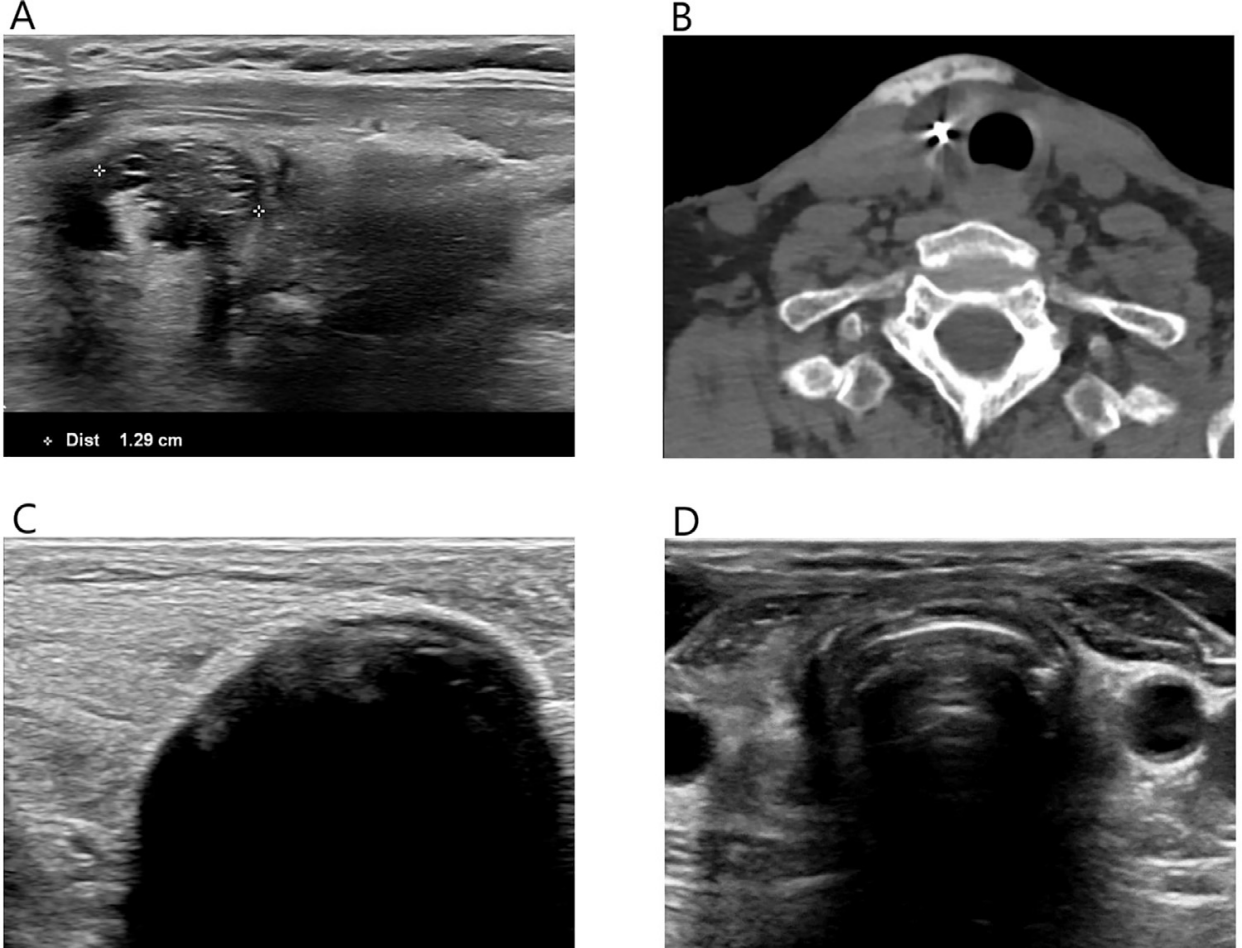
Treatment of recurrent Hurthle cell carcinoma. (A) Preprocedural ultrasound showing the local recurrence in the right thyroid bed. (B) Attempts at hydrodissection between the lesion and the trachea were unsuccessful due to adhesions, yet hydrodissection was successfully performed to protect the vessels and skin. This CT image also shows the cryoablation probe. (C) During cryoablation, the ice ball was expanded to achieve a 2 mm margin beyond the lesion. (D) Follow-up ultrasound after 3 months showed complete involution of the residual lesion.
